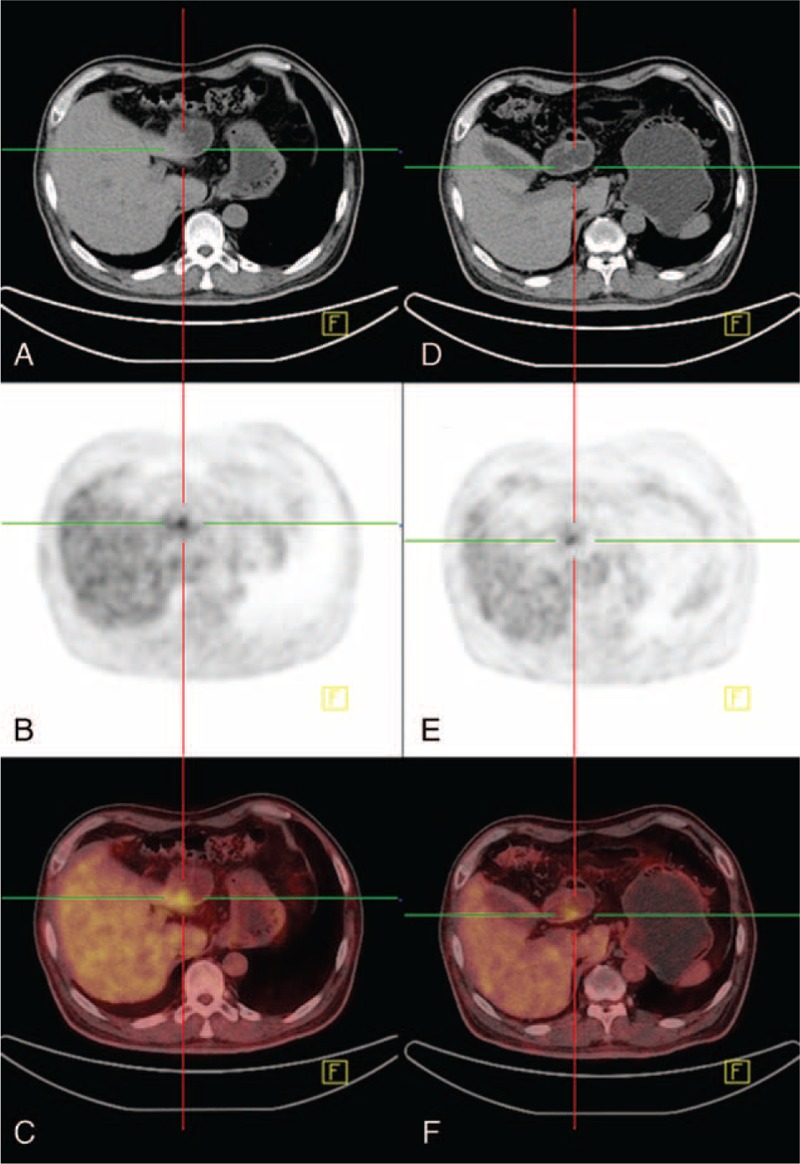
A 58-year-old man was found focally increased 18F-FDG uptake in gastric antrum on PET/CT health check, S1 was 5.2 (A, CT image; B, PET image; C, PET/CT fusion image) on conventional imaging at 60 minutes after tracer injection. Delayed imaging (D, CT image; E, PET image; F, PET/CT fusion image) was performed 65 minutes later, and S2 was 4.7. Both scans showed gastric wall thickening in local gastric antrum. Gastric endoscopy suggested superficial gastritis.
